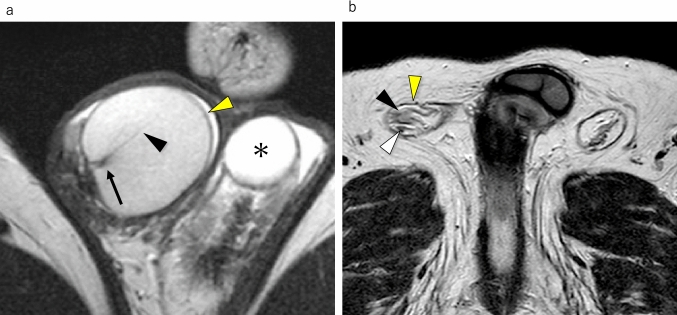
Normal anatomy of the scrotum on MRI. a Axial T2-weighted MR image shows a right testis enveloping tunica albuginea (yellow arrowhead) recognizing as a low-signal structure. Within the testis, the septa (black arrowhead) separating the lobules are observed as linear low-signal structure, continuous to a low-signal structure called the testicular mediastinum (black arrow). In the left scrotum, a spermatocele (asterisk) is observed. b Axial T2-weighted MR image shows the spermatic cords. The spermatic cord is a cord-like structure that contains nerves, blood vessels (black arrowhead), and the vas deferens (white arrowhead). The spermatic cord is enveloped by a layer (yellow arrowhead) comprising the cremasteric muscle sandwiched between internal and external spermatic fasciae
